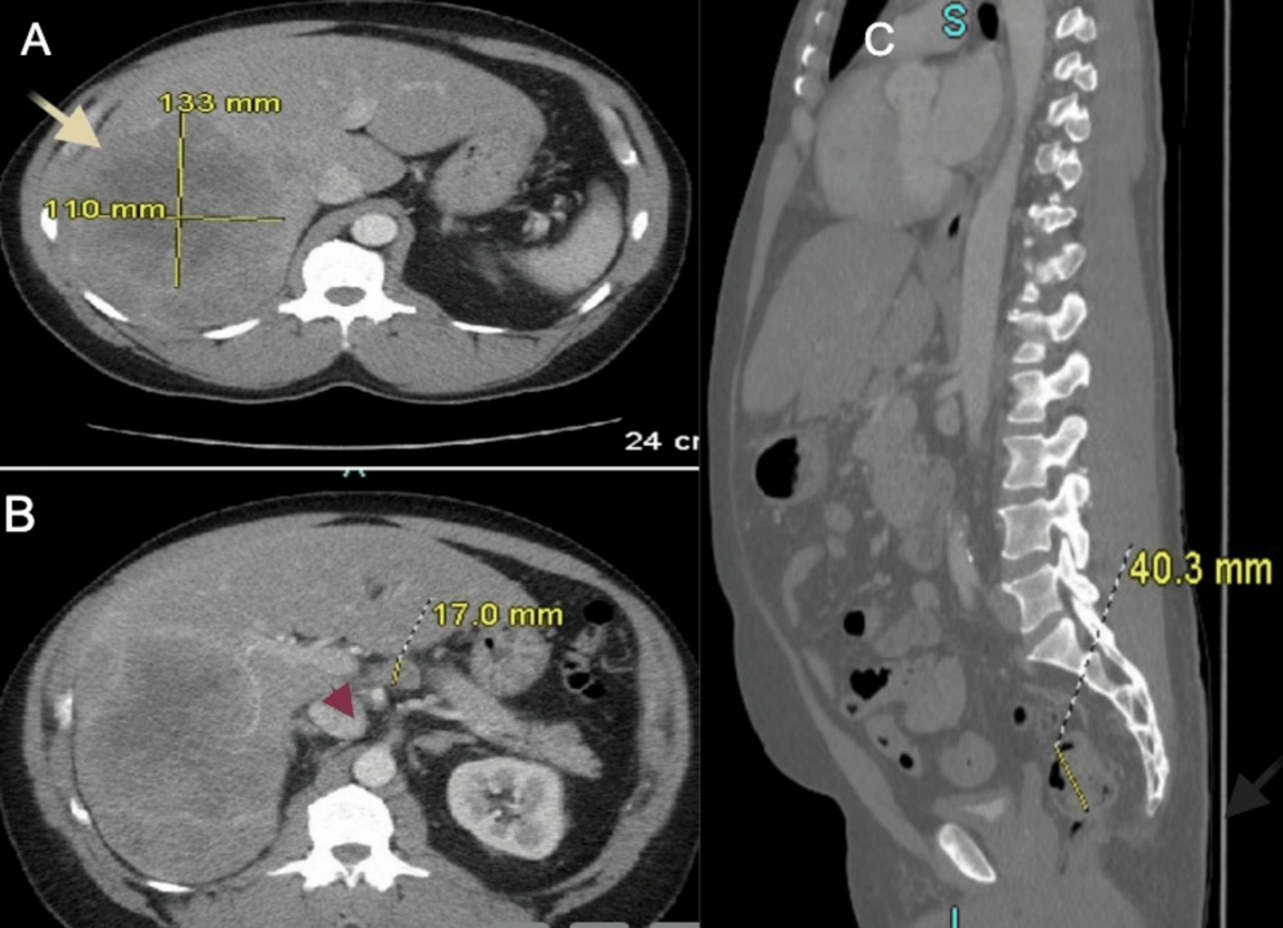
CT scan of the abdomen and pelvis Multiple masses in the right hepatic lobe (A) along with porta hepatis lymphadenopathy (B), both highly suggestive of metastatic disease. Findings in the rectum raised concerns for eccentric mural thickening and nodularity extending to the rectosigmoid junction (C) CT: computed tomography
